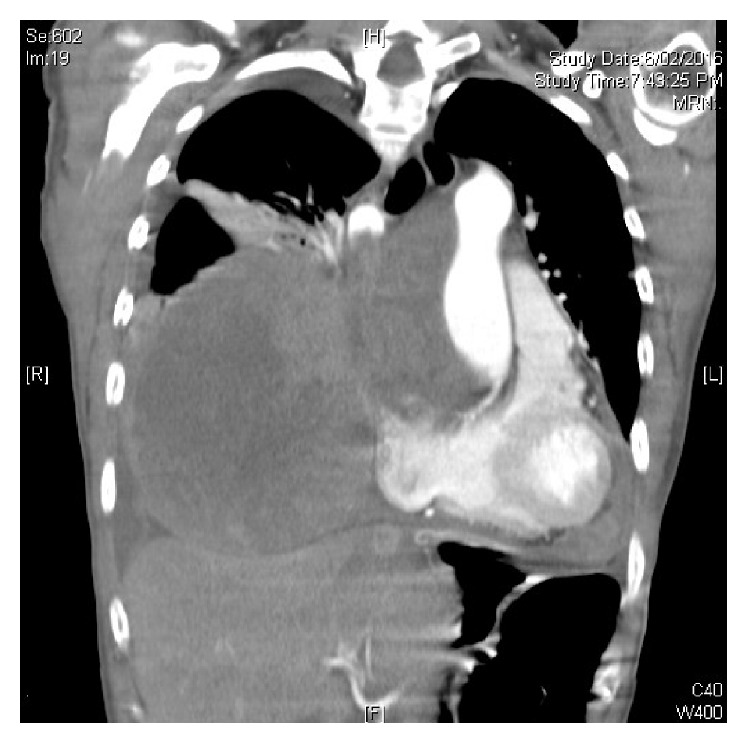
CT coronal 3 months following diagnosis demonstrating progression of disease at the Right atrium and Right lung.
